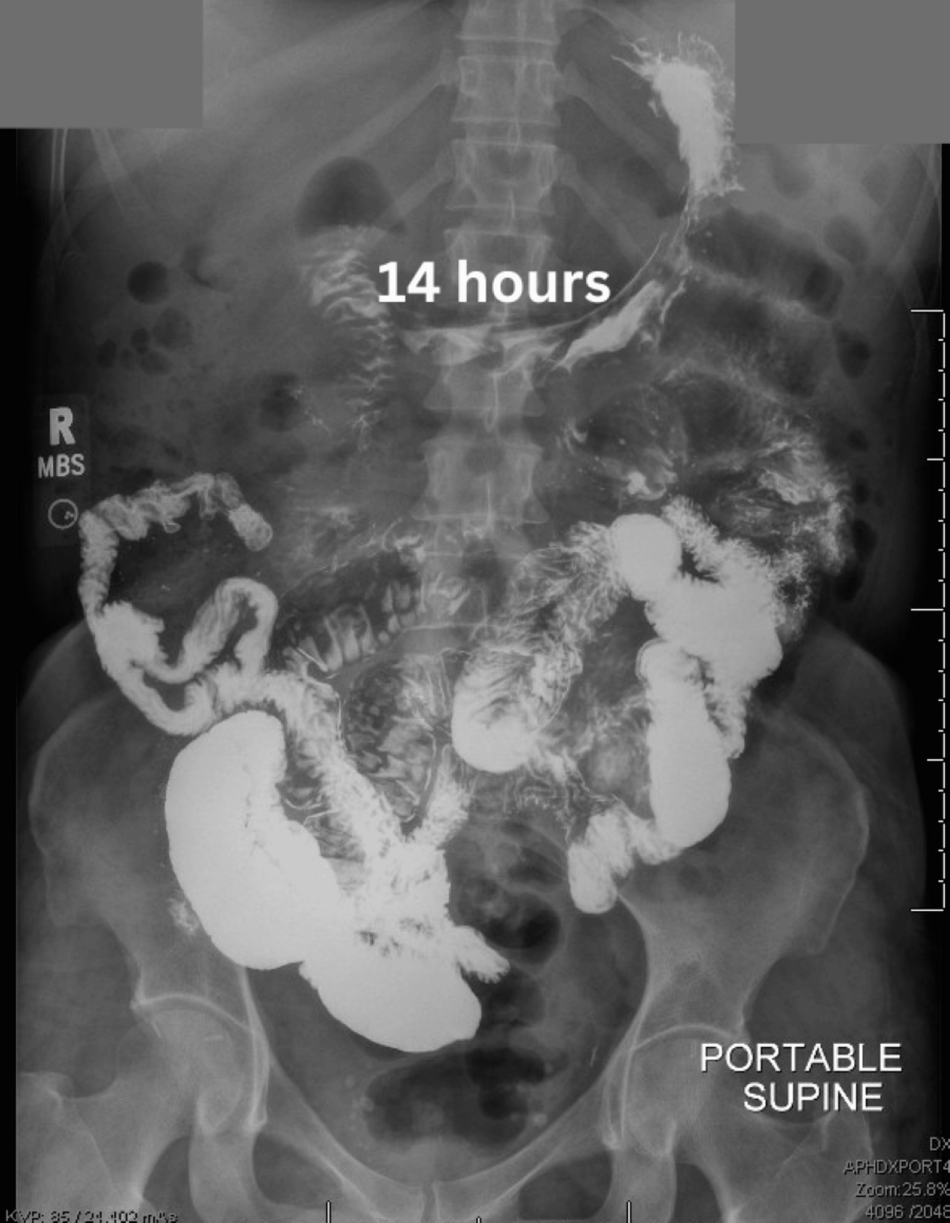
Abdominal radiograph demonstarting barium contrast within the distant small intestines and proximal large intestine at 14 hours
